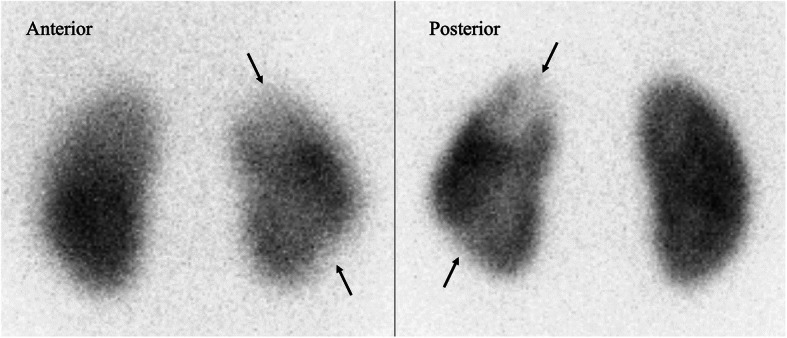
Dimercaptosuccinic acid (DMSA) scan obtained after 4 months from the onset of the disease. The decrease in the renal uptake of radionuclide in the upper and lower poles of the left kidney along the low-density areas in the initial CT scan suggested renal scarring (arrows)
